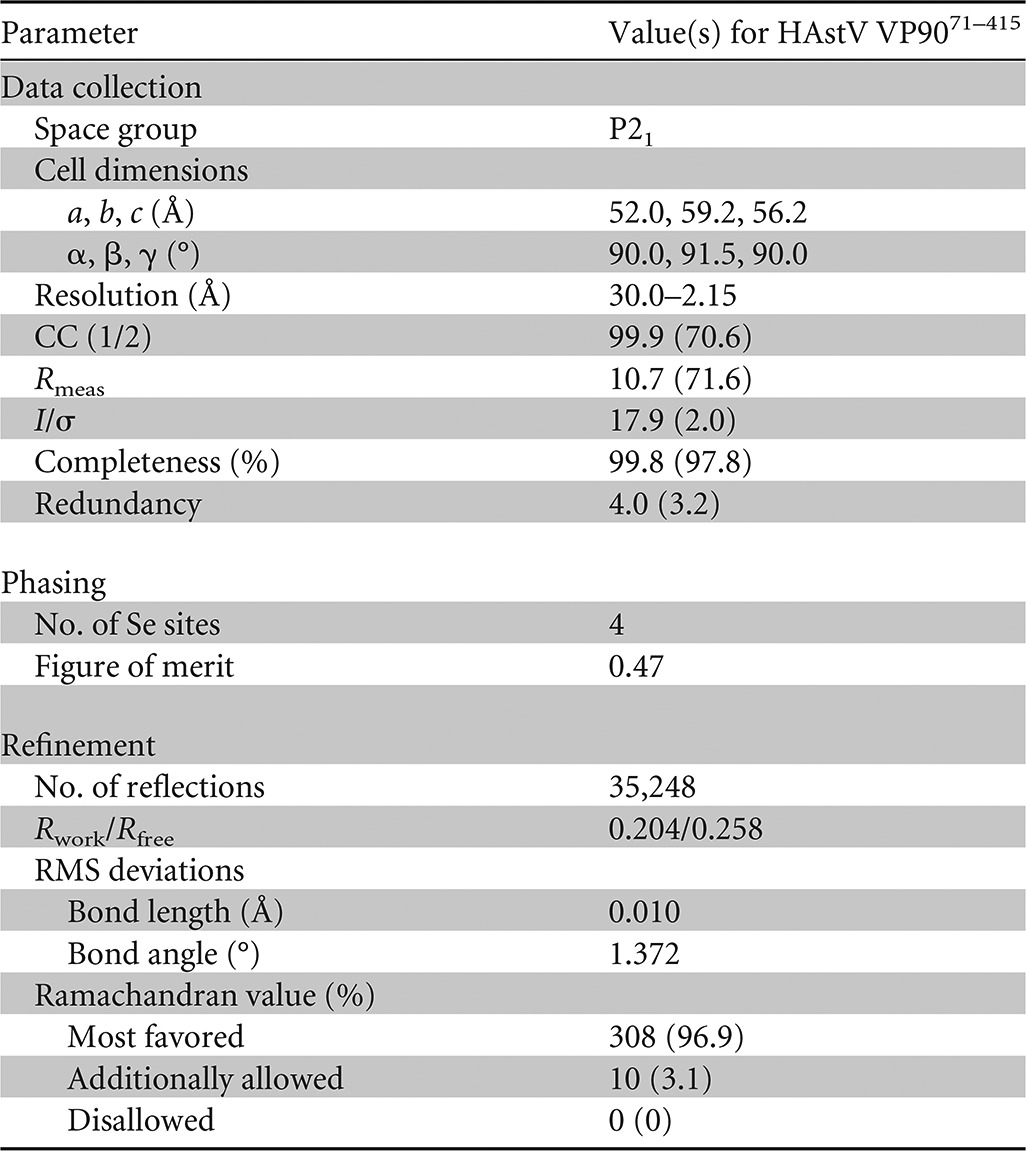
X-ray data statistics^*a*^
